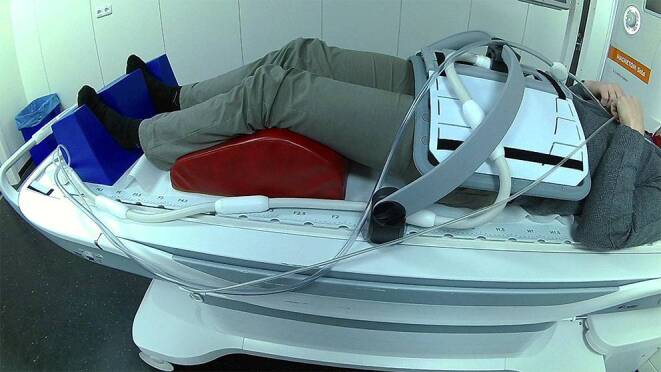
Setup for MR imaging in treatment position for patients with pelvic tumors. The *red *leg rest and the *blue* foot rest are identical to the ones used during radiotherapy treatment delivery. The coil holder in combination with the flat indexable tabletop allows for reproducible positioning
